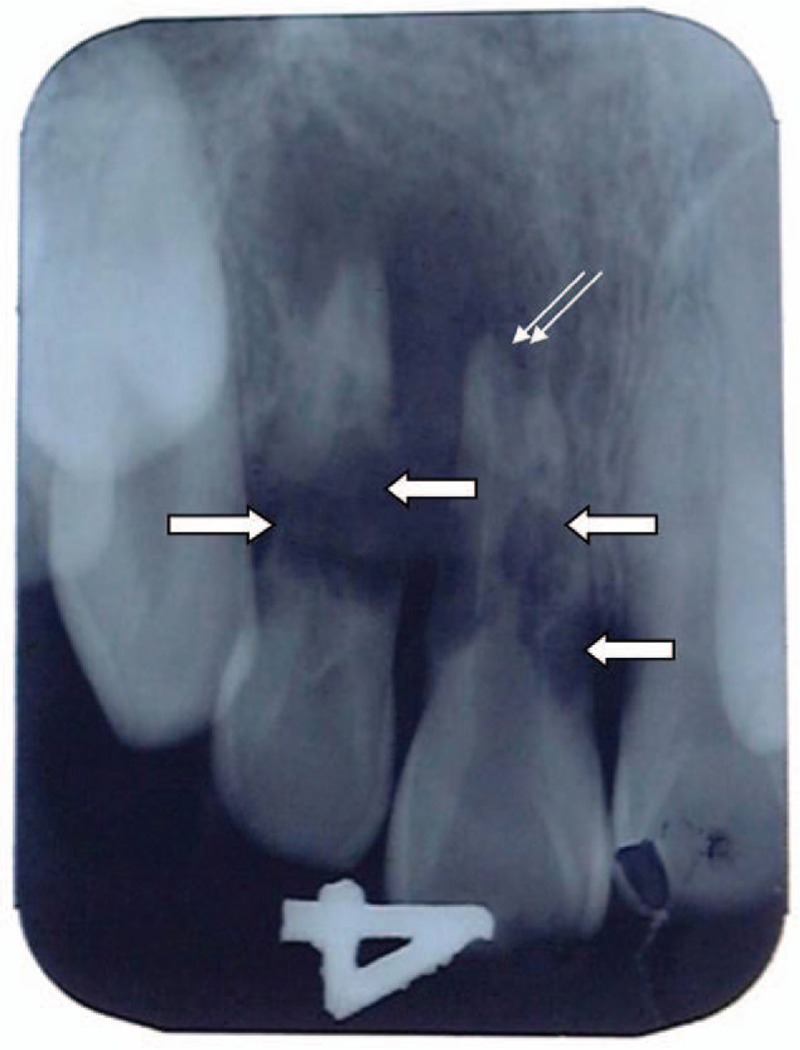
Periapical radiograph of replanted upper left maxillary central and lateral incisors. Several lacunae (bold arrows) are seen in the roots of both the central and lateral incisors. Central incisor shows periapical involvement (double arrow), whereas no radiolucency was observed around the periapical region of the later incisor.
